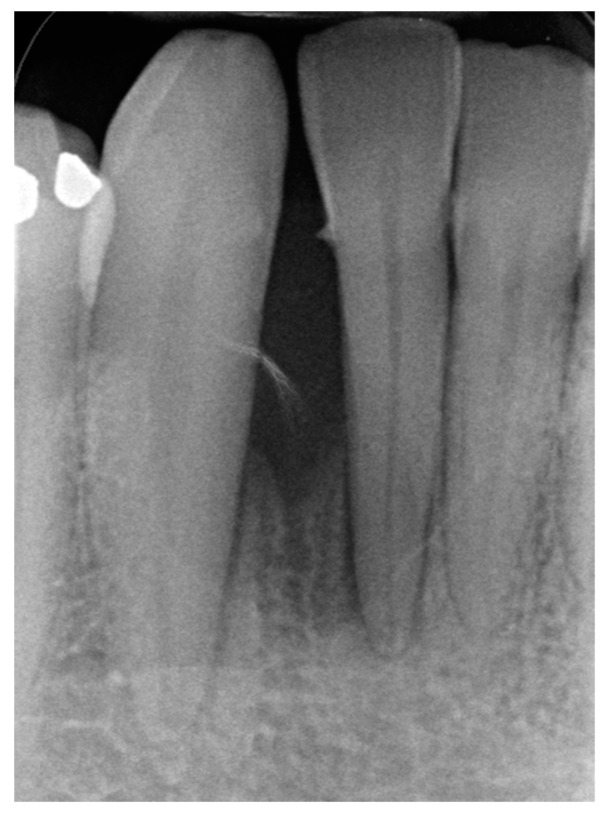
Resorption of alveolar bone surrounding and root deviation of lateral incisors as evident in dental X-ray.
